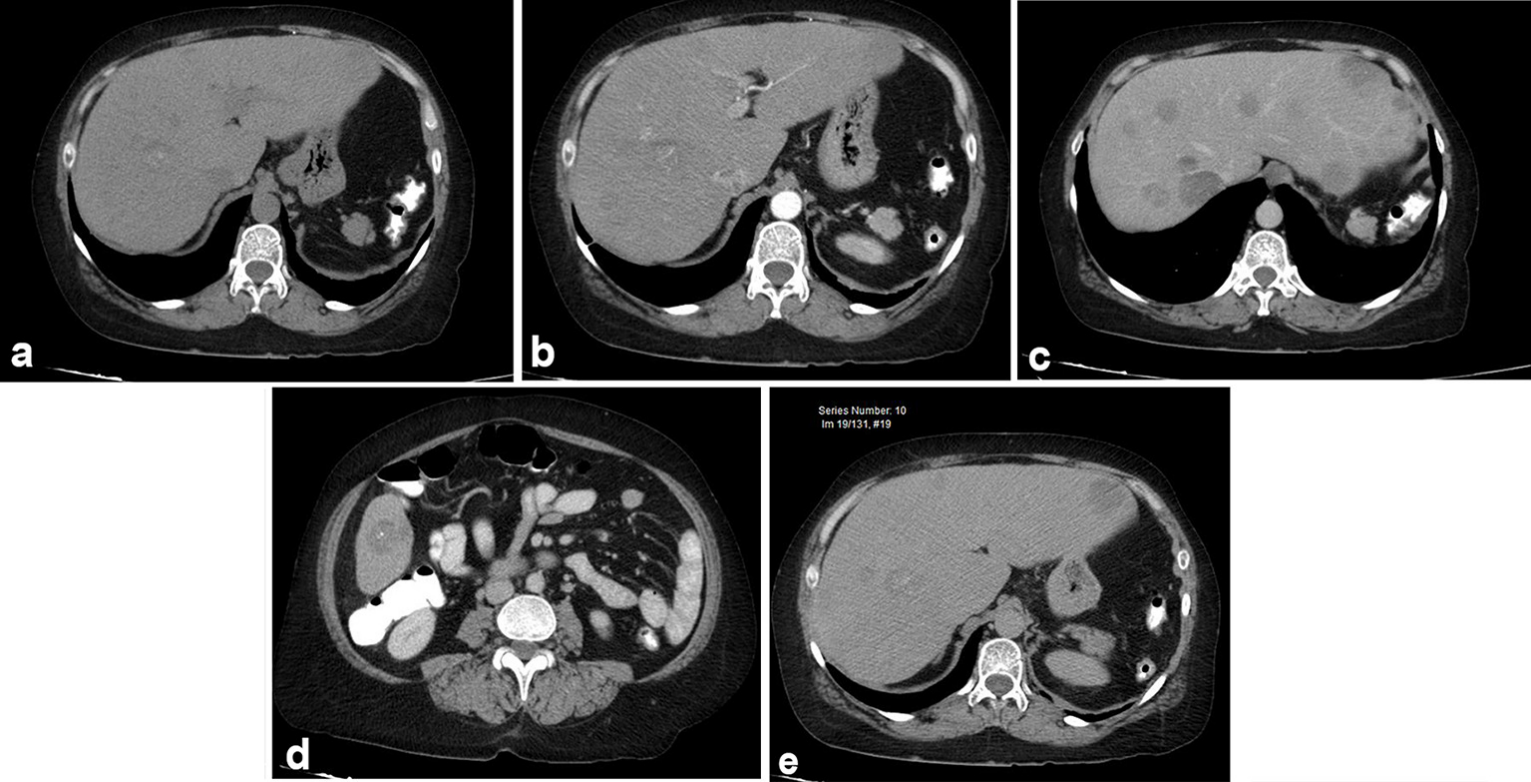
Abdominal CT showing multiple hepatic lesions ranging from a few to 5 cm, which do not significantly enhance and contain microcalcifications. (a) Simple phase: focal lesions are hypodense with microcalcifications (arrow); (b) arterial phase, without significant enhancement: no hypervascular lesions are identified. (c and d) Portal phase: discrete uptake; (e) late phase: persisting hypodense lesions without retention of contrast medium.
